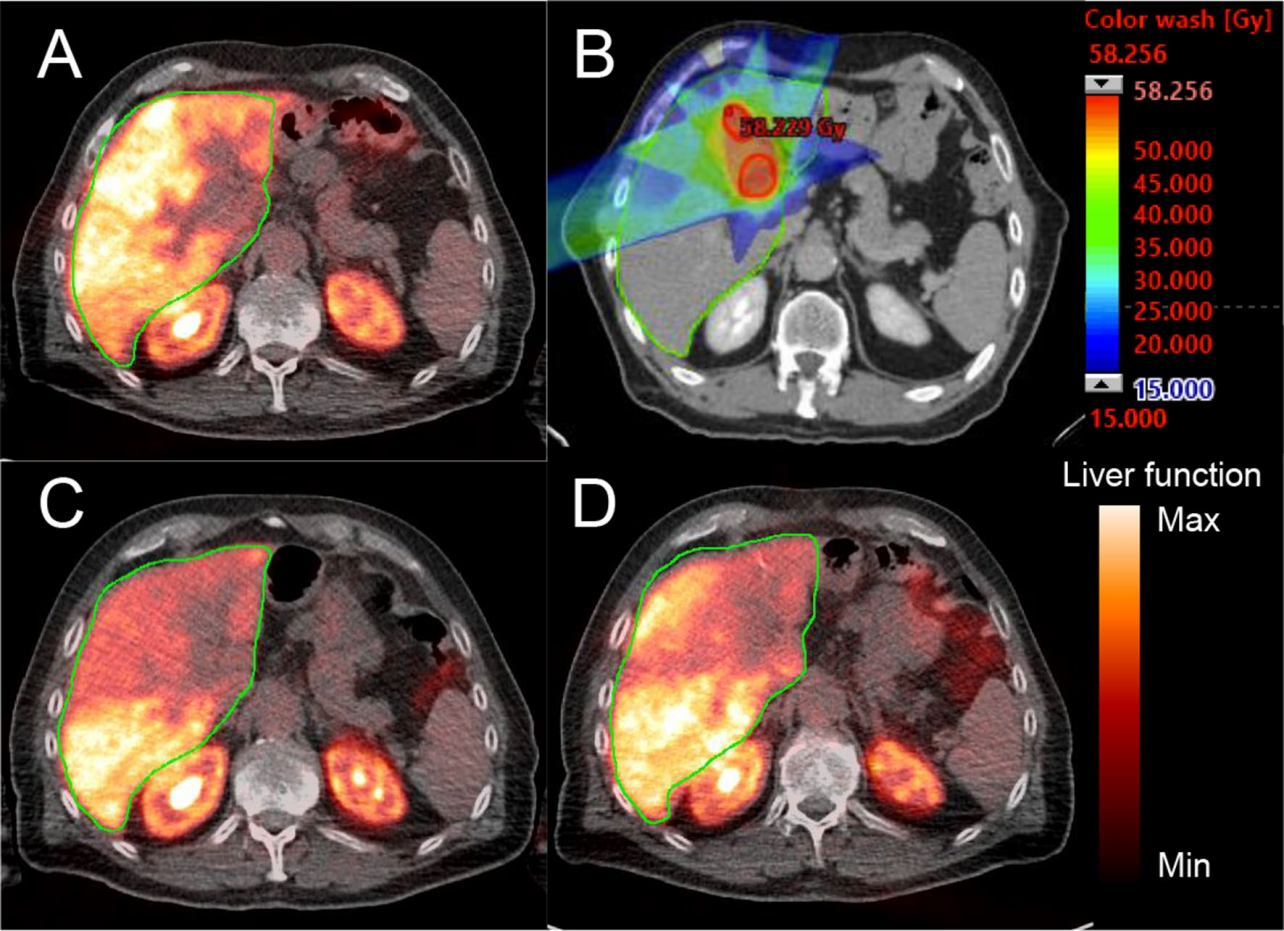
Example of parametric image of *K*_met_ (hepatic systemic clearance of [^18^F]FDGal, mL blood/min/mL blood). A is before SBRT of a colorectal liver metastasis; note that the tumour does not accumulate [^18^F]FDGal. B is the treatment planning CT with dose color wash of 15 Gy. C and D show parametric images after 1 month and 3 months, respectively. The liver is encircled by a green line and the color scale for the PET images show *K*_met_ ranging from 0.0 to 0.3 mL blood/min/mL liver tissue
